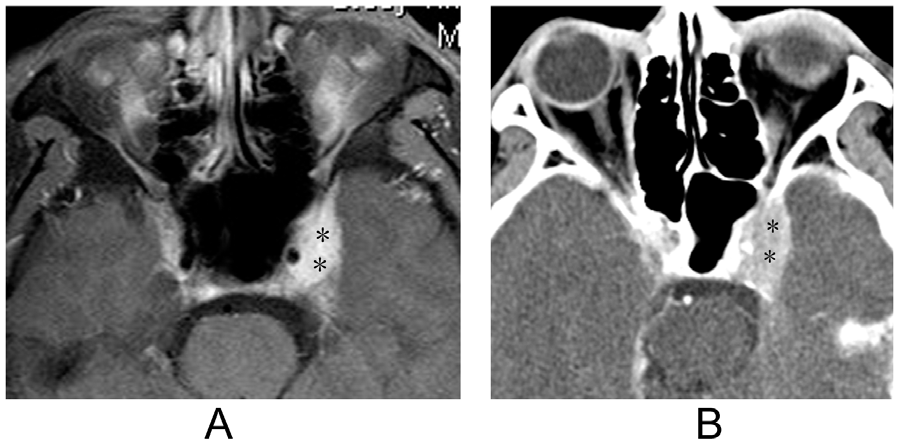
Idiopathic orbital inflammation syndrome with cavernous sinus involvement. Figure 4A: Axial fat-saturated contrast-enhanced T1w imaging reveals a lesion that extends into the left cavernous sinus (asterisks). Figure 4B: Axial contrast-enhanced CT imaging reveals enlargement of the left cavernous sinus. The radiological contours of the enlargement are similar to those observed with MR imaging (asterisks).
